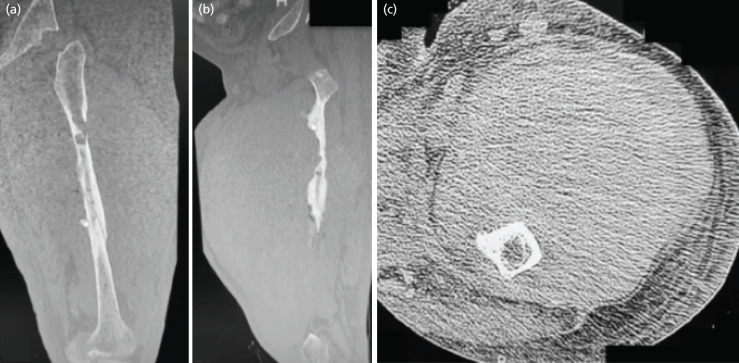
(a) CT scan representative images of the left femur in anteroposterior, (b) sagittal (B), and (c) axial views, noting erosion of the cortex, as well as a large mass on the anterior compartment of the thigh.
